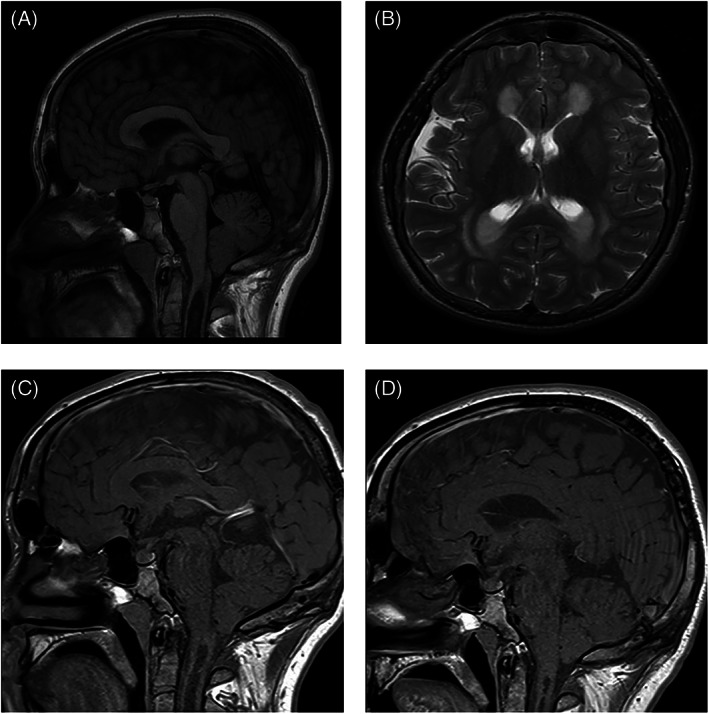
Sagittal T1 MRI of male patient diagnosed by HRS as a neonate aged 16 demonstrates mild inferior herniation of the cerebellar tonsils with a syrinx of the cervical cord (A) and hyperintensity of the white matter and corpus striatum on Axial T2 MRI in keeping with GA type I (B). Follow‐up midline sagittal T1 images shows interval improvement of the cervical cord syrinx aged 22 (C) and 27 (D) years.
